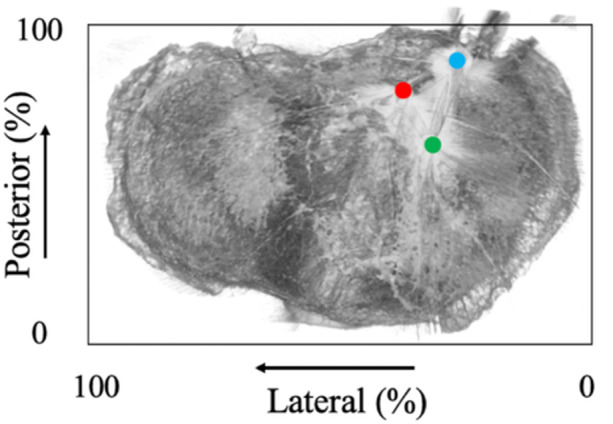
Evaluation of the bone tunnel position using three‐dimensional (3D) computed tomography (CT) of the tibial plateau: anatomical (red dot), anterior (green dot) and posterior (blue dot) bone tunnels are shown.
